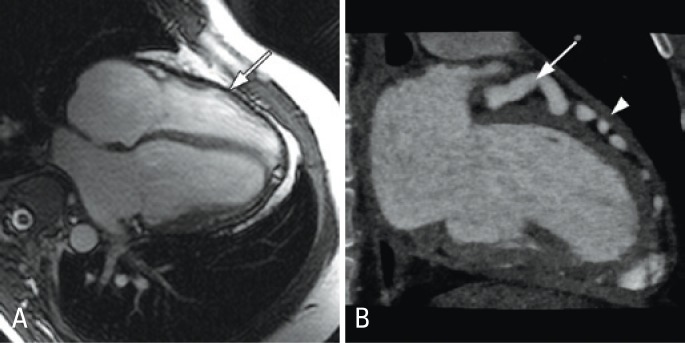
(A) Cardiac magnetic resonance imaging showing pericardial thickening (arrow). (B) Dilated left anterior descending artery (arrow) and thickened pericardium (arrowhead) as demonstrated by cardiac computed tomography
